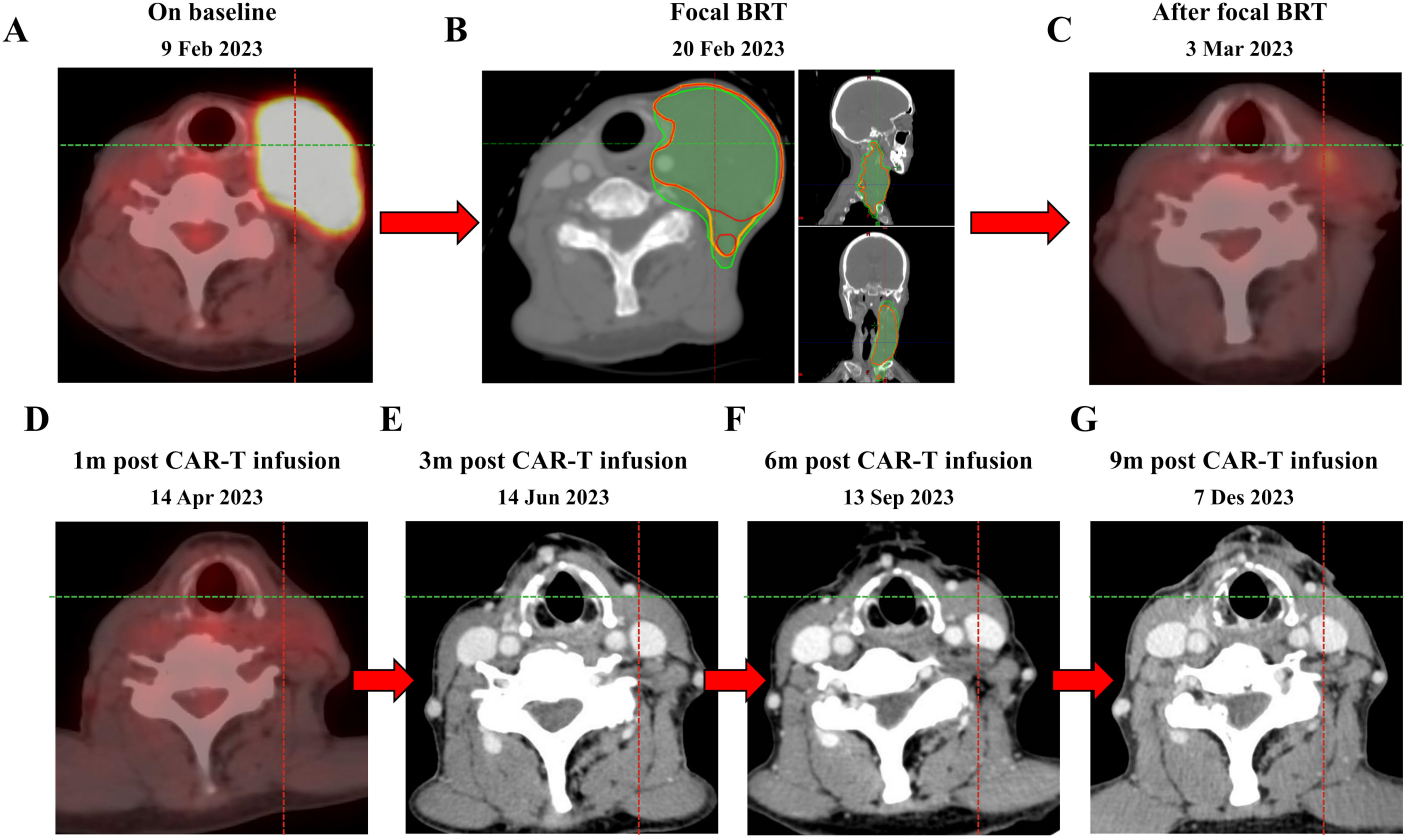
PET-CT scan before bridging radiotherapy (9 Feb 2023) (A), bridging radiotherapy treatment (20 Feb 2023) (B) and after bridging radiotherapy treatment (3 Mar 2023) (C). The patient remains in CR with CAR-T therapy to date (D–G). bridging radiotherapy, bridging radiotherapy. GTV, gross tumor volume. CTV, clinical tumor volume. PTV, planning target volume. The red line represents GTV, the yellow line represents CTV, and the green line represents PTV.
